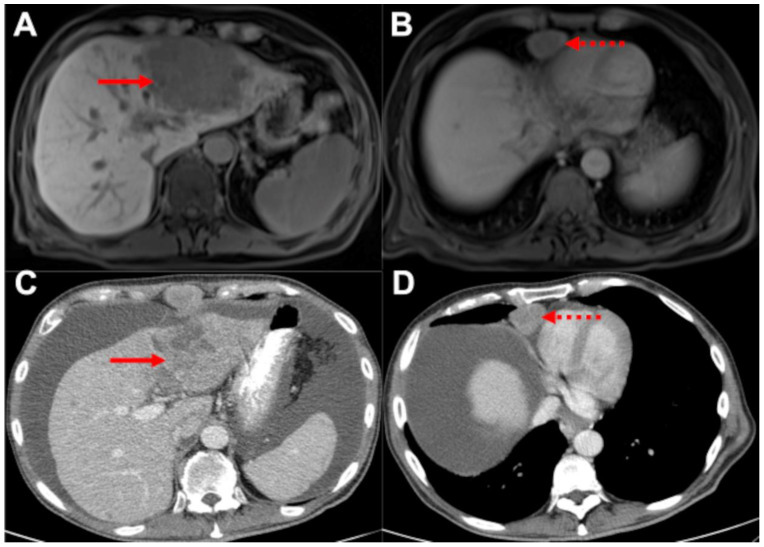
No change in management as EHD is present in both MRI and CT. (A,B): liver MRI, hepatobiliary phase, and axial plane. (C,D): abdomen and chest CT, soft tissue window, axial plane. Both MRI (A,B) and CT (C,D) show the HCC in liver segment II (solid arrows) and a cardiophrenic lymph node metastasis (dashed arrows). Therefore, palliative treatment was recommended, and additional CT staging did not result in a change in patient management. Paracentesis was performed between CT and MRI.
